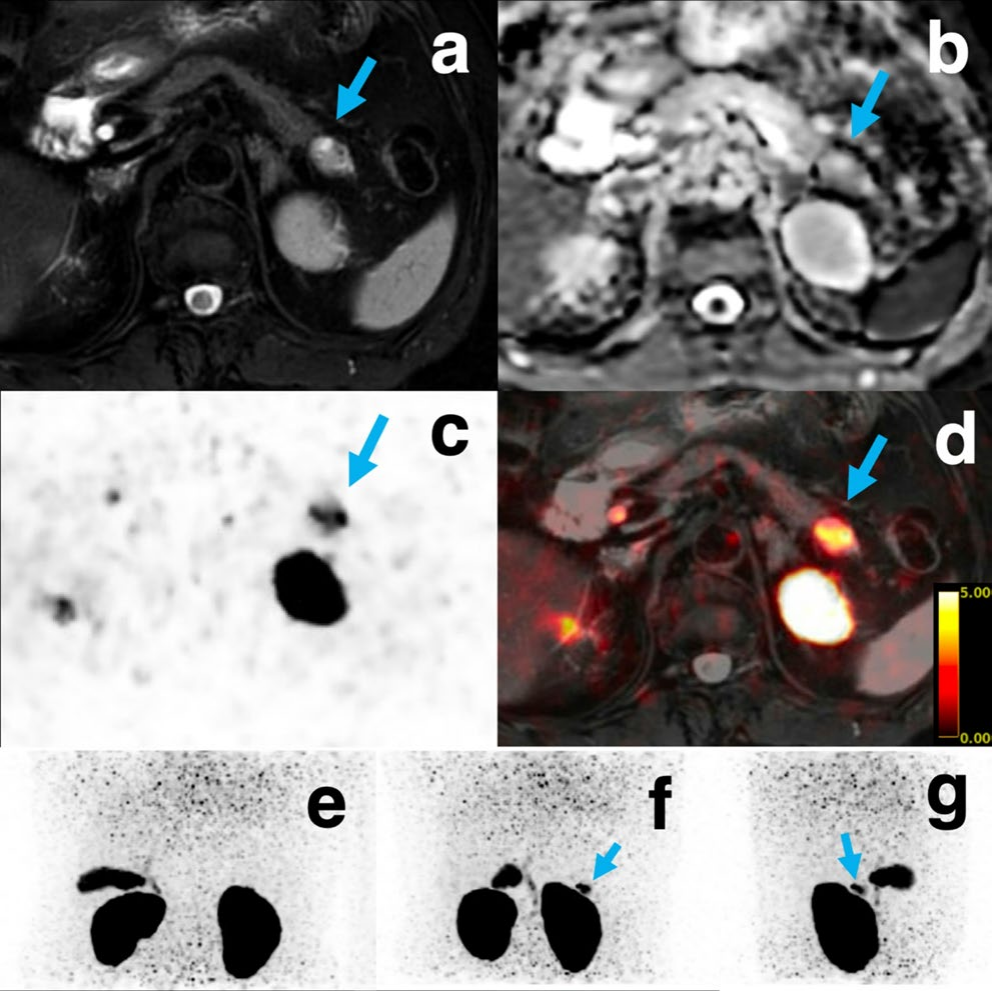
Insulinomas are rare, typically small pancreatic neuroendocrine tumors that often elude detection on widely used imaging methods like computed tomography (CT), magnetic resonance imaging (MRI), and somatostatin receptor (SSTR)-based positron emission tomography (PET), owing to their small size and low levels of SSTR expression [1]. Technically demanding procedures like endoscopic ultrasound and selective arterial calcium stimulation testing can improve sensitivity, but their invasive nature may preclude clinical use [2]. Conversely, because most benign insulinomas display increased levels of glucagon-like peptide-1 receptors (GLP-1R), we developed a PEGylated exendin‑4 probe labeled with [^18^F]FB(ePEG12)12‑exendin‑4 (^18^F-exendin-4)—that enables both qualitative and quantitative diagnosis [3–5].
